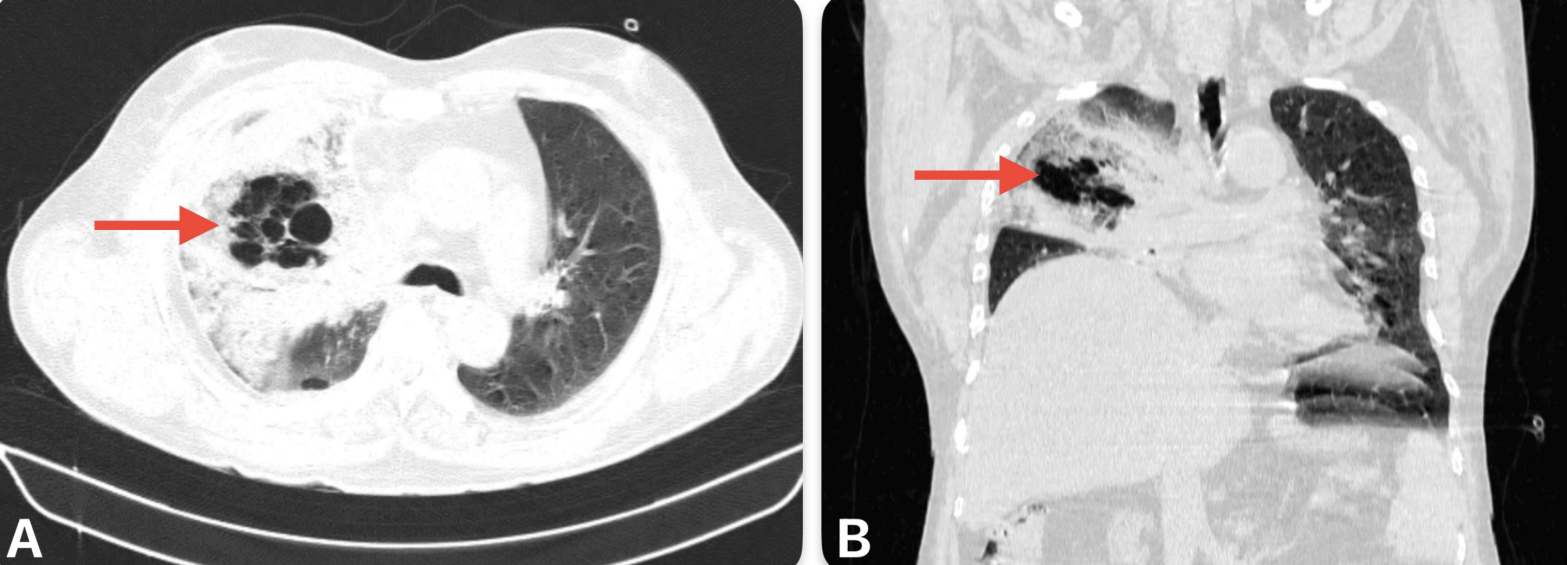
CT imaging of thorax lung window. (A) Axial view showing bubbly cystic lucencies with surrounding ground-glass opacity. (B) Coronal view showing surrounding consolidation.
